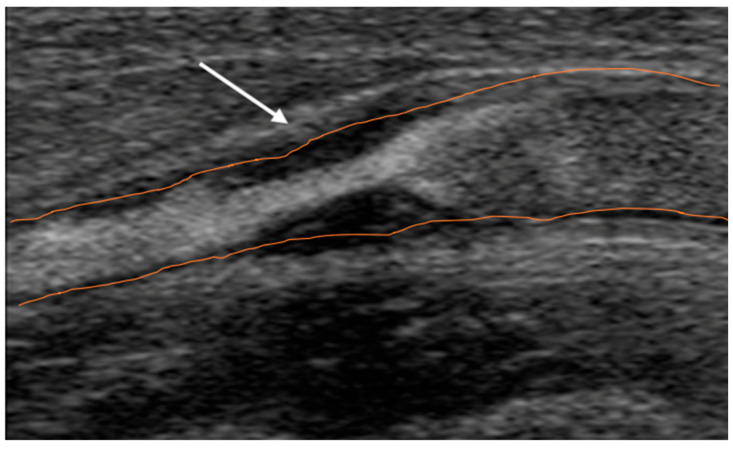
B-mode Doppler ultrasound image of carotid stenosis (arrow).
